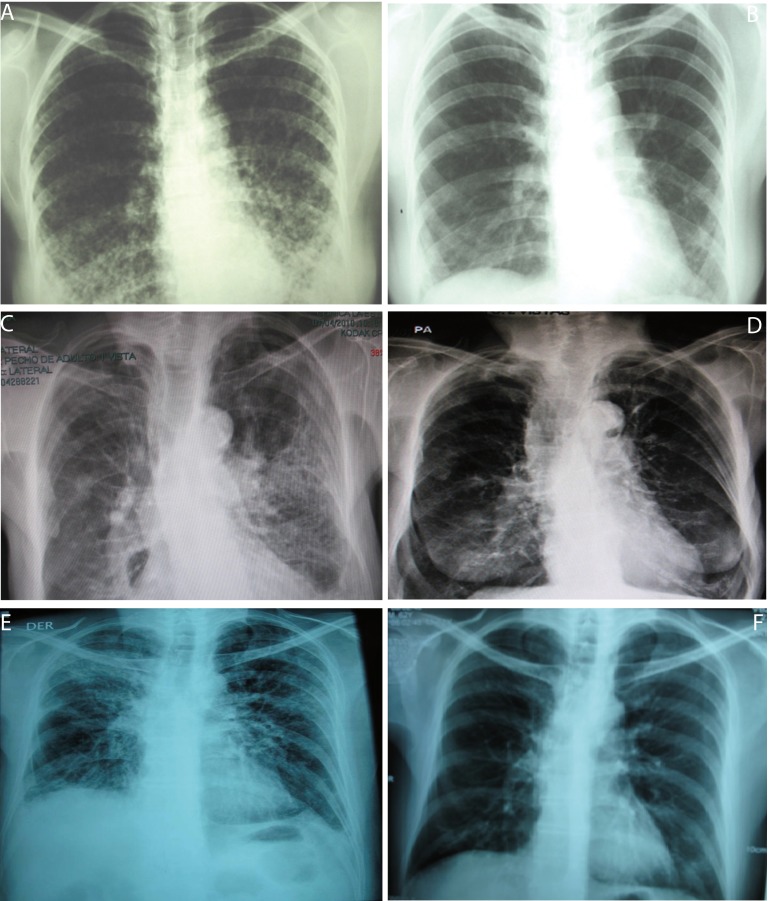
Chest X-ray of 3 patients. A and B: Chest X-ray of patient 1, December 2001 and July 2003 before and 22 months after ganciclovir treatment end, respectively. C and D: Chest X-ray of patient 5, April 2010 and September 2010, before and two months after ganciclovir-vaganciclovir treatment end, 4 months after trimethoprin sulfamethoxazole treatment end and 1 month after voriconazol treatment end, respectively. E and F: Chest X-ray of patient 6, November 2009 and February 2010, before and two months after trimethoprin sulfamethoxazole end and at ganciclovir treatment end, respectively.
